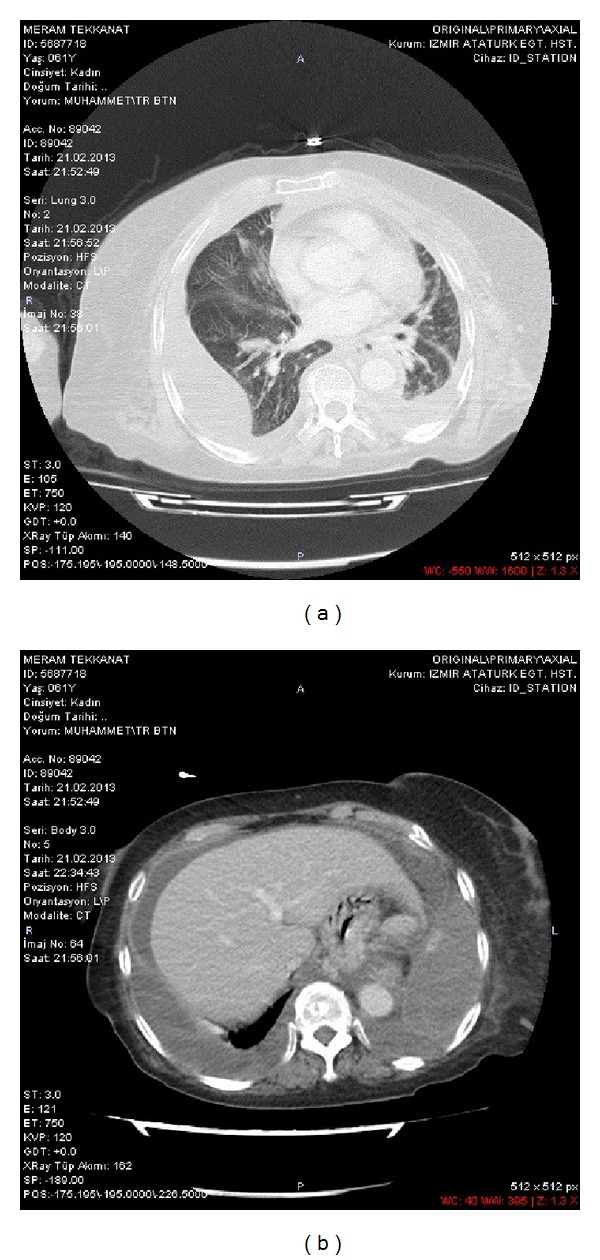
(a) A chest CT scan revealed bilateral pleural effusion with a partial compression at the left lung. (b) An abdomen CT scan revealed large amount of ascites of chylous type.
